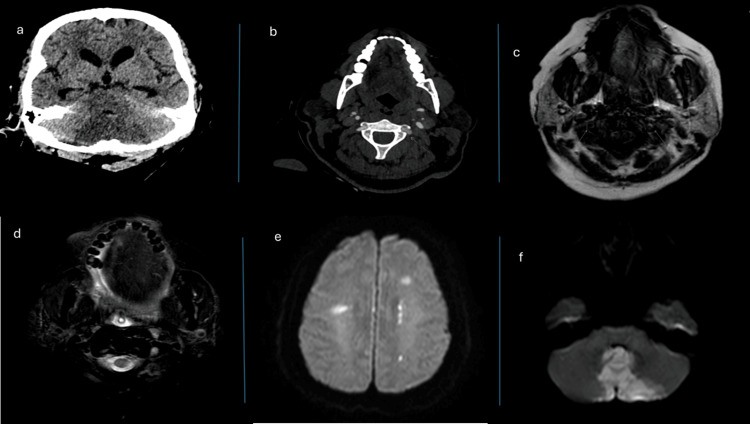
CT carotid angiogram and four MRI of a patient with spontaneous vertebral artery dissection (a) Unenhanced CT scan at the time of presentation demonstrating cerebellar hypodensity along the posterior superior cerebellar artery. (b) Faint opacification of the left vertebral artery with subtle linear filling defect. (c) and (d) Axial T1 and T2 demonstrate loss of normal signal void at the left vertebral artery on T2 with high T1 signal abnormality consistent with vertebral artery dissection. (e) and (f) Corresponding diffusion-weighted images shows showering emboli and multiple areas of restricted diffusion at the cerebral white matter with cerebellar infarcts along the distribution of posterior inferior cerebellar artery and perforators.
